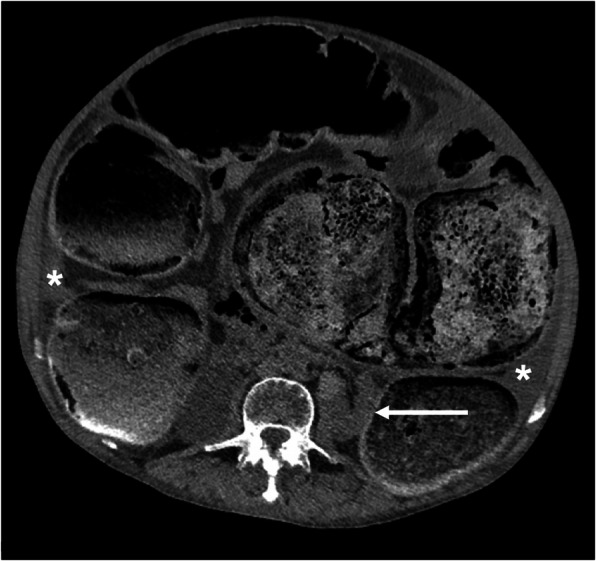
Axial view of the abdominal computed tomography revealing a marked backward compression of the kidneys (white arrow) together with aperitoneal effusion (*)
